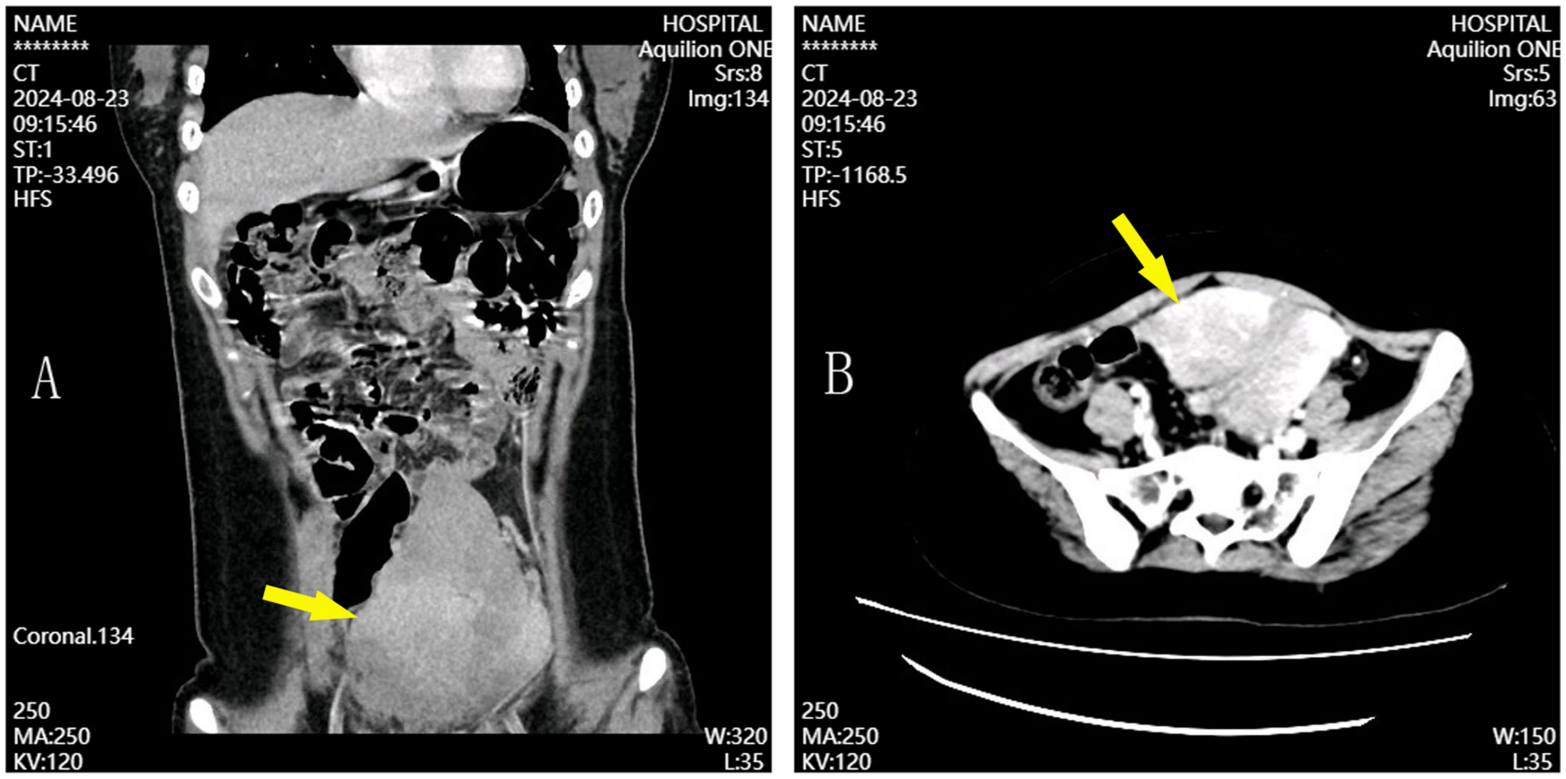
Coronal (A) and axial (B) CT scans showing splenic displacement and hypoperfusion consistent with infarction.
